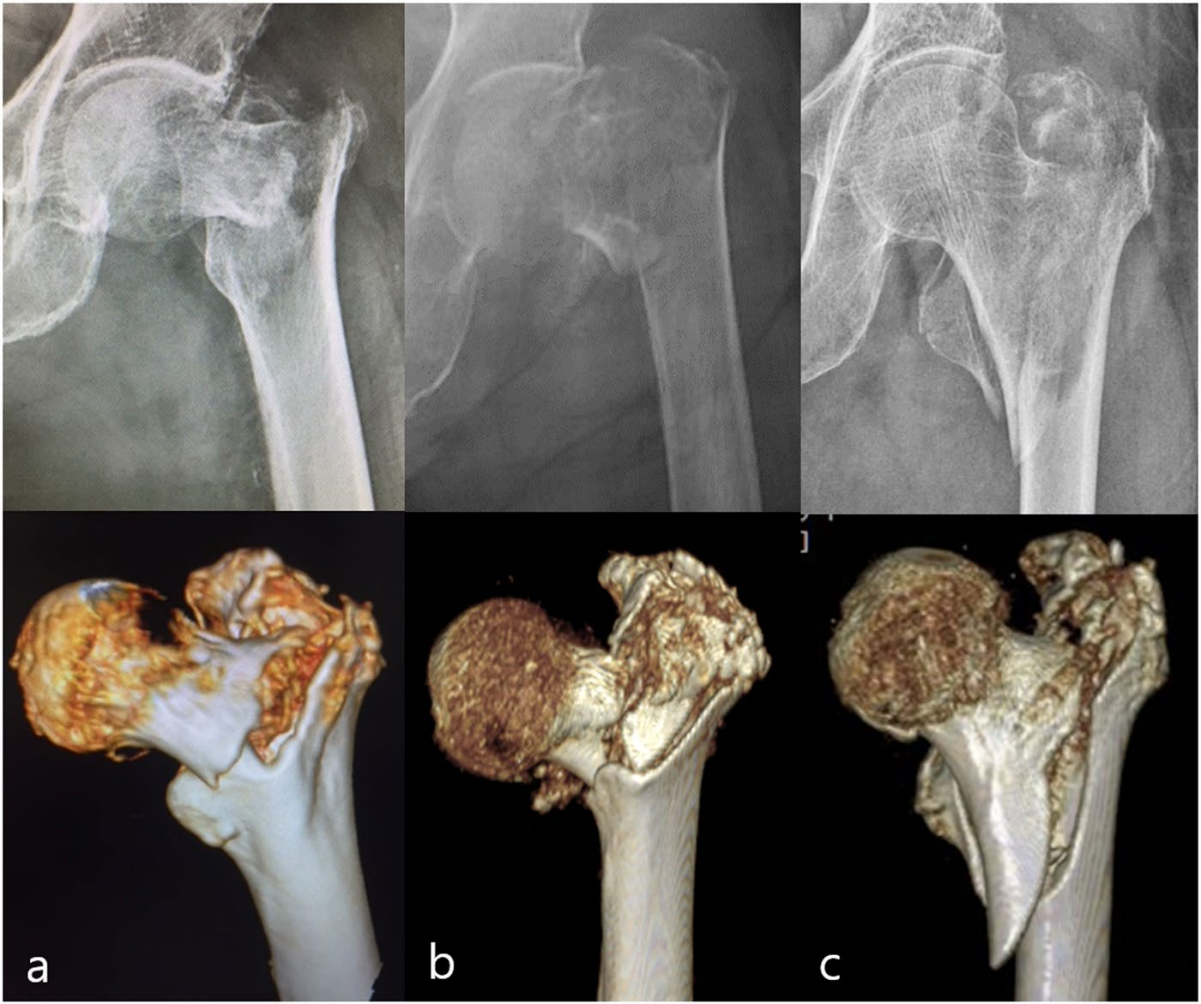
(a) Basicervical fracture component, (b) comminution of the anterior cortex, and (c) comminution of GT confirmed on preoperative radiographs and 3-D CTs.
